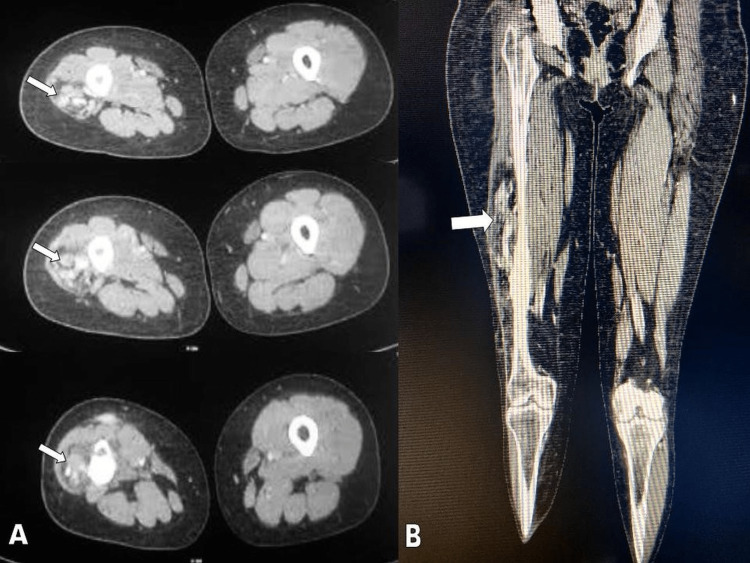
CT venogram A circumscribed heterodense intramuscular mass within vastus lateralis muscle measuring 2.0 (anteroposterior) x 4.5 (craniocaudal) x 3.5 (transverse) cm, with multiple enhancing tortuous vessels (white arrows) is seen in the axial plane of the right thigh (A). Coronal plane (B) showed diffuse atrophy of muscles of the proximal thigh with regional fatty replacement of vastus lateralis muscle (white arrow) CT: computed tomography
